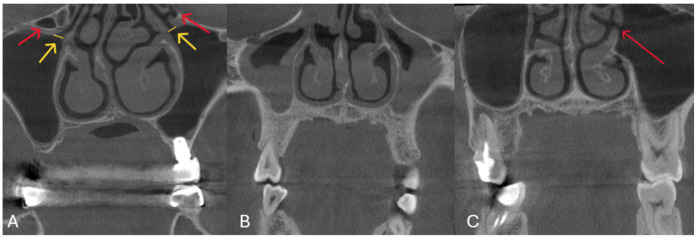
Coronal section of a CBCT image. (A): The maxillary ostium is indicated by yellow arrows, and HCs are indicated by red arrows and yellow lines indicate ostium width; (B): coronal section of a CBCT image demonstrating ostium obstruction; (C): coronal section of a CBCT image showing the accessory maxillary ostium indicated by a red arrow.
